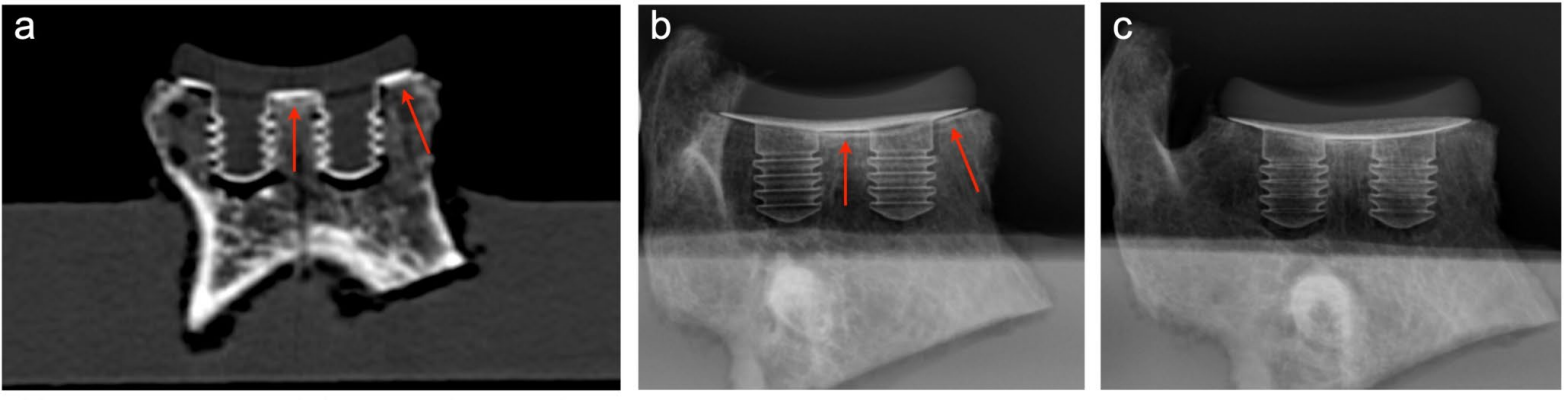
Different quantities in bone support after implantation surgery in the CT scan and on conventional X-rays (a) CT scan of the coronal cutting plane shows no gap between the coated PE glenoid and bone. (b) Conventional true a. p. radiograph displaying a DL in zones 3 and 5. (c) -10-degree craniocaudal (cc) tilted a. p. radiograph showing no signs of RLL or DL
